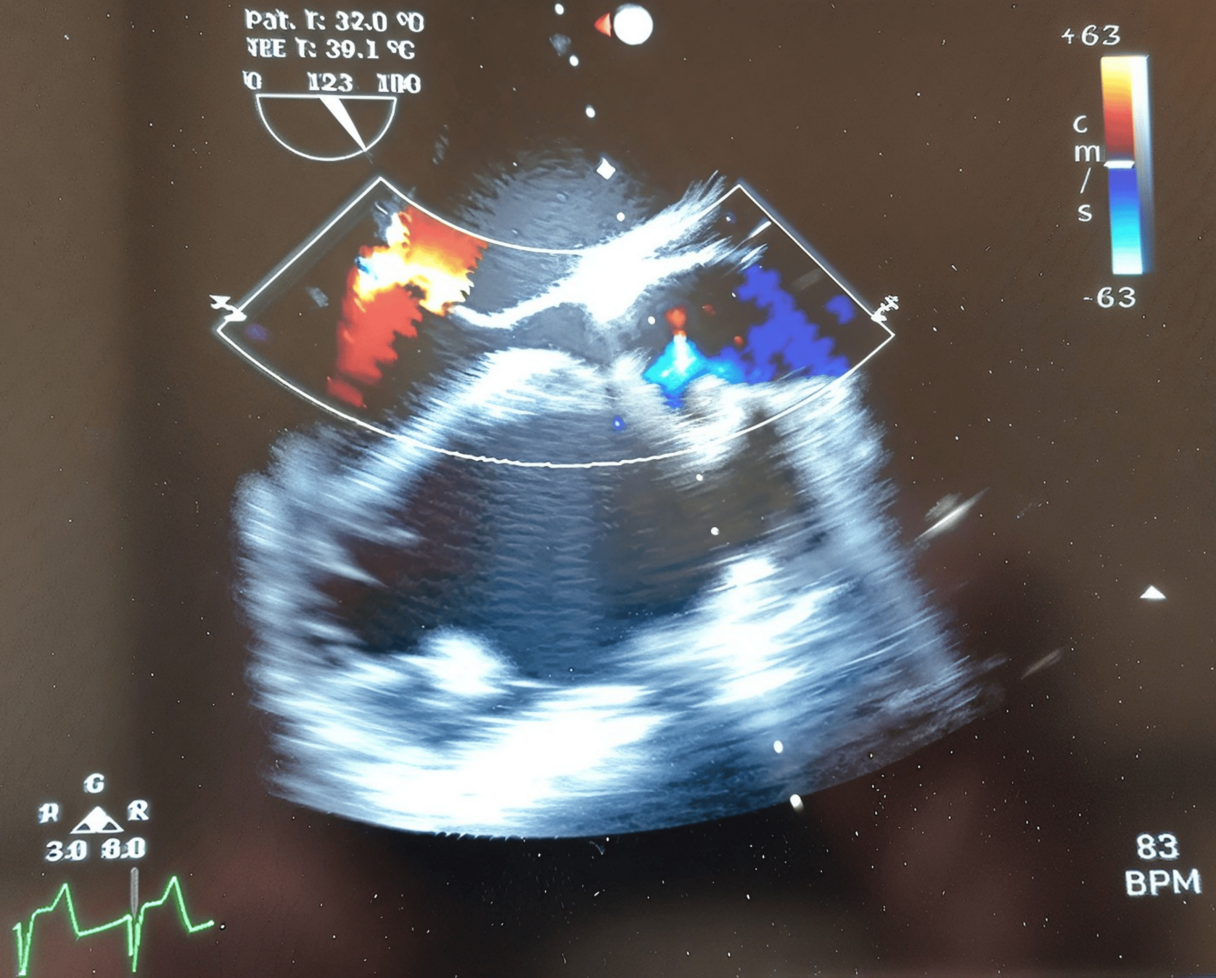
Aortic valve short axis with aortic regurgitation (as observed in our perioperative transesophageal echocardiography).
